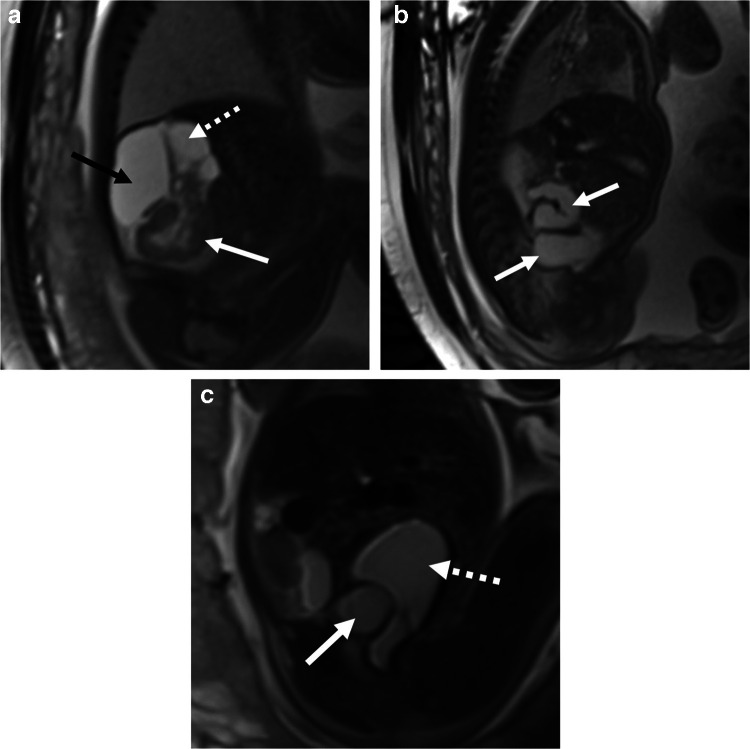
MRI imaging of a 30-week female fetus demonstrating a duplex kidney with upper pole moiety obstruction. a Sagittal Single-Shot Fast Spin Echo (SSFSE) image showing normal renal parenchyma at the lower pole moiety (*white arrow*), dilation of the upper pole collecting system with cystic obstructive dysplasia of the parenchyma (*dotted white arrow*), and a larger posterior fluid collection, likely urinoma (*black arrow*). b Sagittal SSFSE image showing the dilated, tortuous upper pole ureter (*white arrows*). c Coronal SSFSE image showing a ureterocele (*white arrow*) at the ureter insertion into the urinary bladder (*dotted arrow*)
